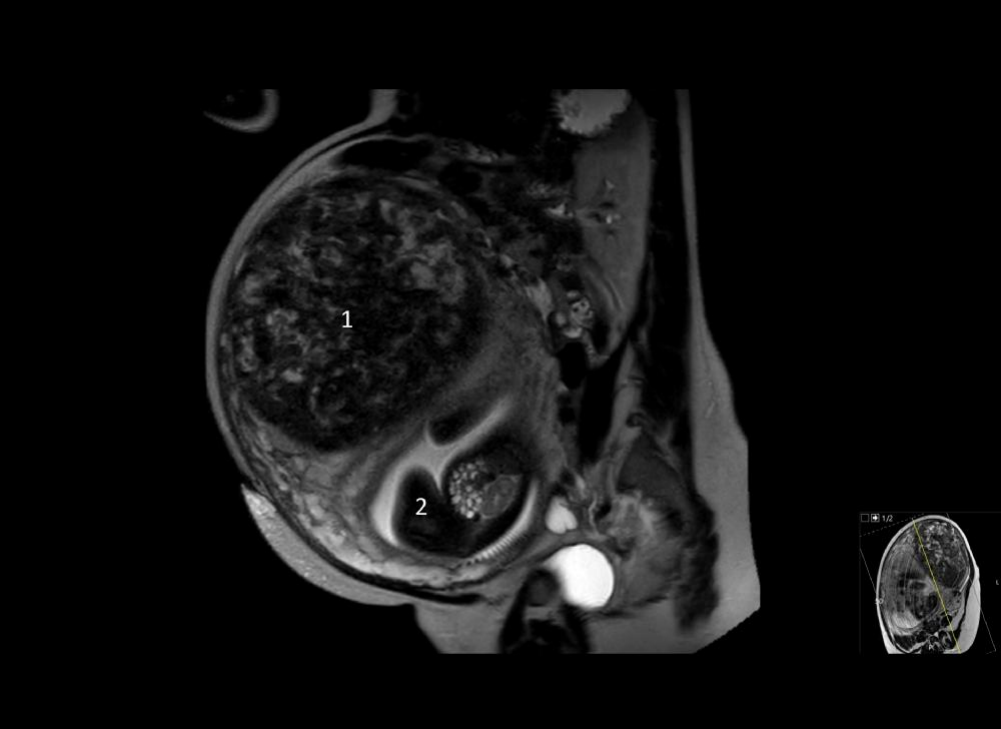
Sagittal magnetic resonance imaging at 31 weeks of pregnancy with uterus myoma (1) 7.9 × 8.7 inches (20 × 22 cm) and fetus dorsocaudal in uterus (2).
